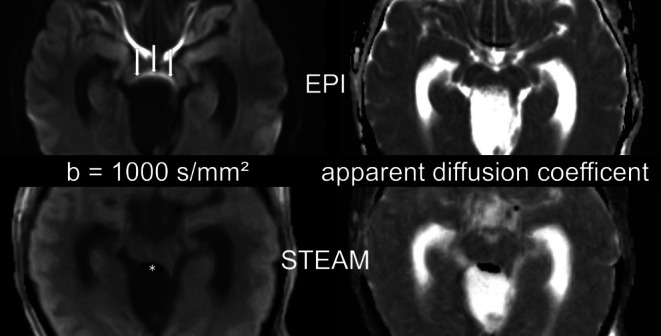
23-year-old female. Postoperative b = 1000 s/mm^2^ images (*left*) and apparent diffusion coefficient (ADC) maps (*right*) obtained by EPI DWI (*top*) and STEAM DWI (*bottom*) 40 h after resection of an infratentorial arachnoid cyst with compression of the brain stem. In EPI DWI only hyperintensities (*arrows*) of the dorsal mesencephalon caused by susceptibility artifacts were noticed. An infarction could not be excluded. In STEAM DWI air (*asterisk*) and the compression of the brain stem can be detected. An infarction of the mesencephalon could be excluded
